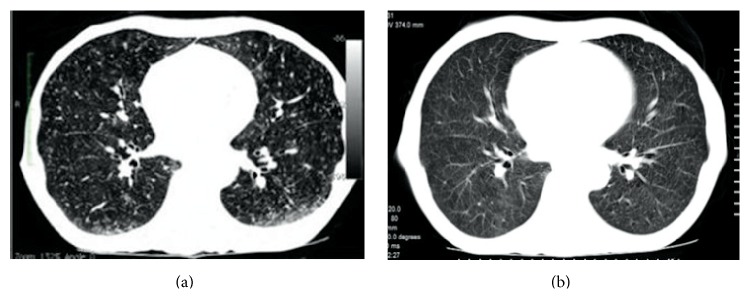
Chest CT images at (a) presentation and (b) fifth month of anti-TB treatment.
